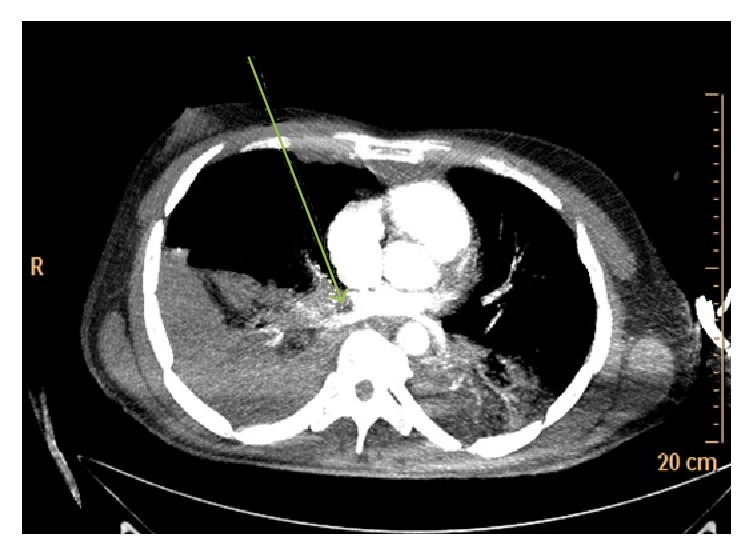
Coronal slice contrast enhanced computed tomography pulmonary angiography. The arrow demonstrates a large filling defect (pulmonary embolus) in the right pulmonary artery with an oligaemic right lung field.
